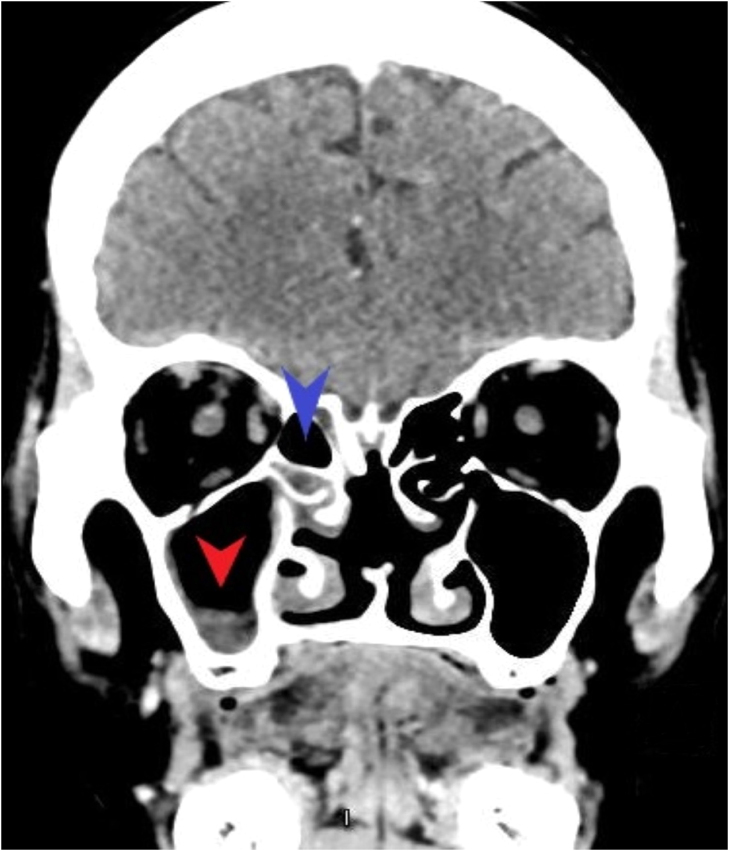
C.T. Head scan with contrast (coronal view) showing partial opacification of the right ethmoid (blue arrow-head) and right maxillary (red arrow-head) sinuses. CT: Computed Tomography.
